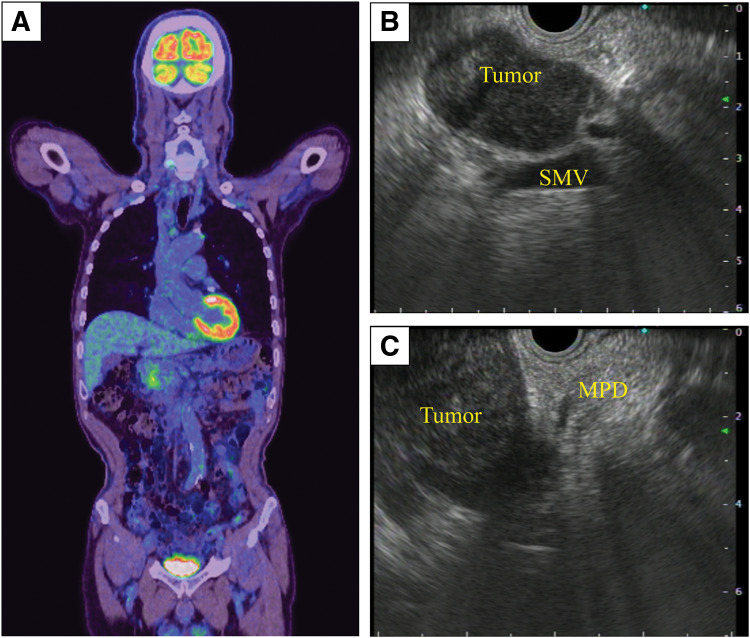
PET-CT and EUS imaging. (A) PET-CT at the initial diagnosis shows increased metabolic activity in the pancreatic head. (B, C) EUS imaging at the initial diagnosis shows that the tumor is contiguous with the pancreas, without invasion into the SMV. EUS, endoscopic ultrasonography; MPD, main pancreatic duct; SMV, superior mesenteric vein
